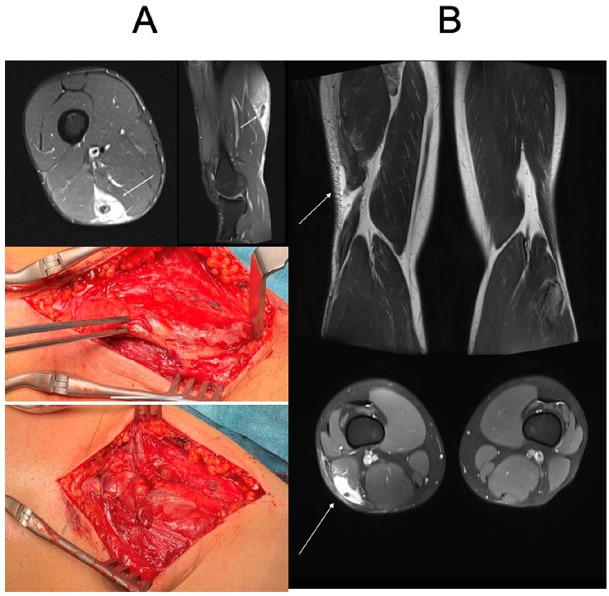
A) Distal tendon rupture of the ST (MRI and perioperative images before and after repair) and B) distal MTJ area rupture of the BF (MRI images, arrows indicate the retracted muscle belly and tendon stump).
